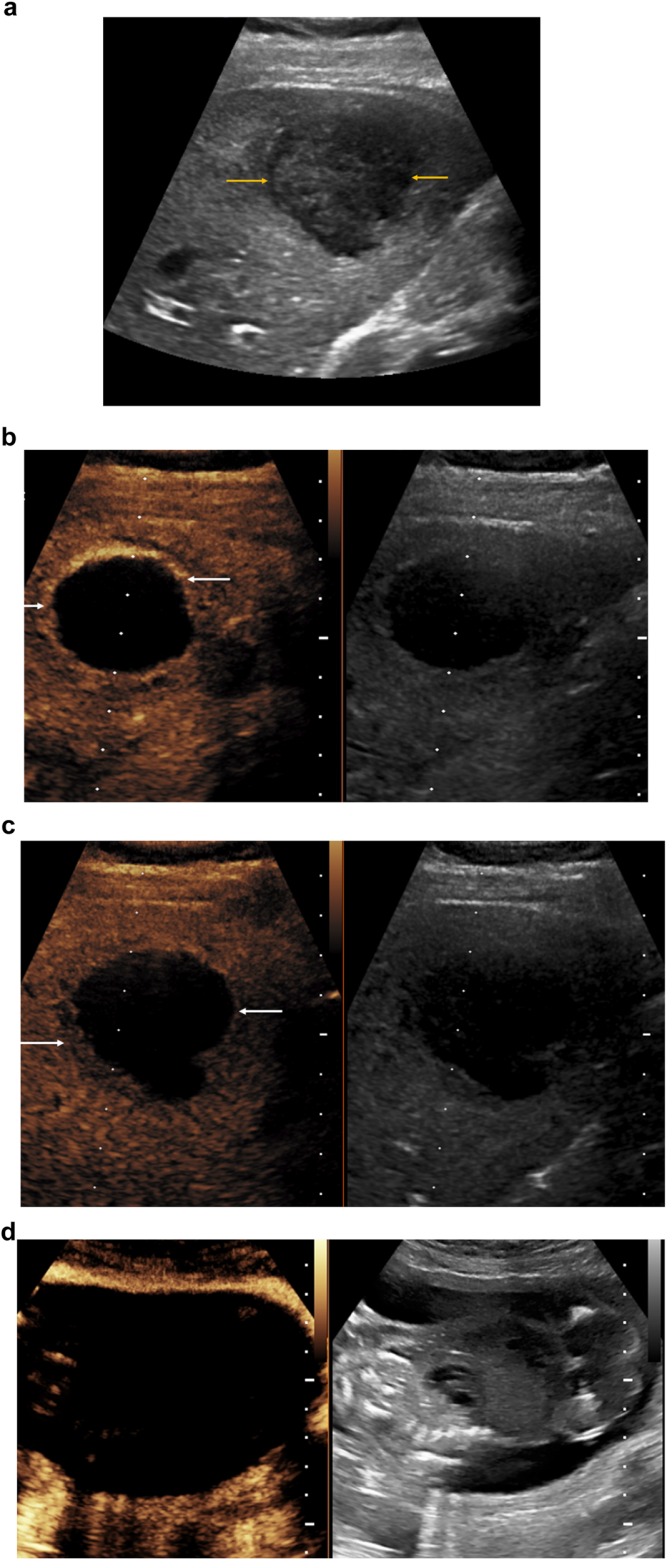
A 29-year-old woman in the eighth week of pregnancy presented with upper abdominal pain. An unenhanced MR examination detected a focal liver lesion, but could not offer a firm diagnosis, with a differential diagnosis of possible necrotic tumour, pyogenic abscess or a hydatid cyst. a The baseline B-mode ultrasound demonstrates the low reflective focal liver lesion (arrows) in the left liver lobe, with no distinguishing features. b Following the administration of 2.4 mL of SonoVue™ (Bracco SpA, Milan) there is no enhancement of the central aspect of the lesion, with peripheral rim enhancement (arrows) typical of an abscess. c On the later phase of the CEUS examination, washout of the rim was noted (arrows). A directed aspiration of the cystic structure was performed with the final diagnosis of amoebic abscess. d The extension of the ultrasound examination to the pregnancy sac, demonstrates no contrast enhancement of the foetus
